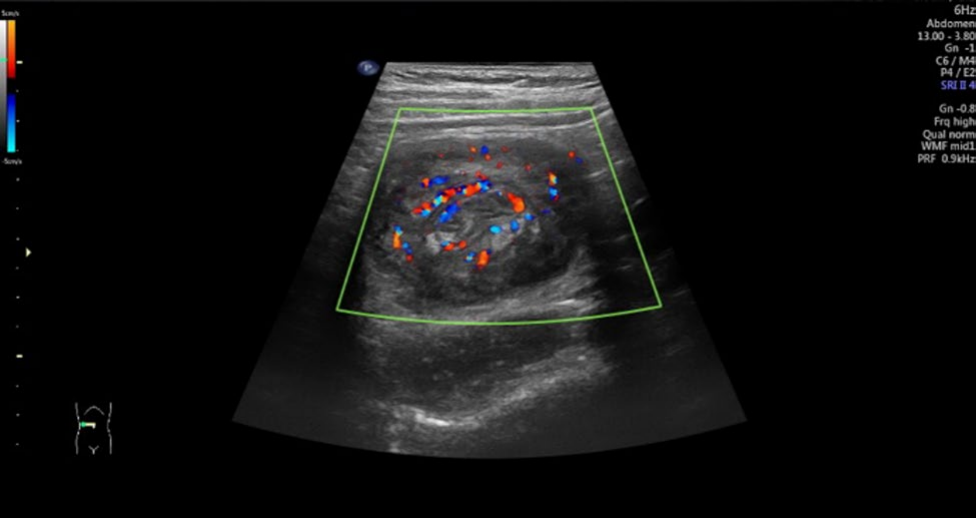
Intussusception Color Doppler Flow Signal (Grade 2)
